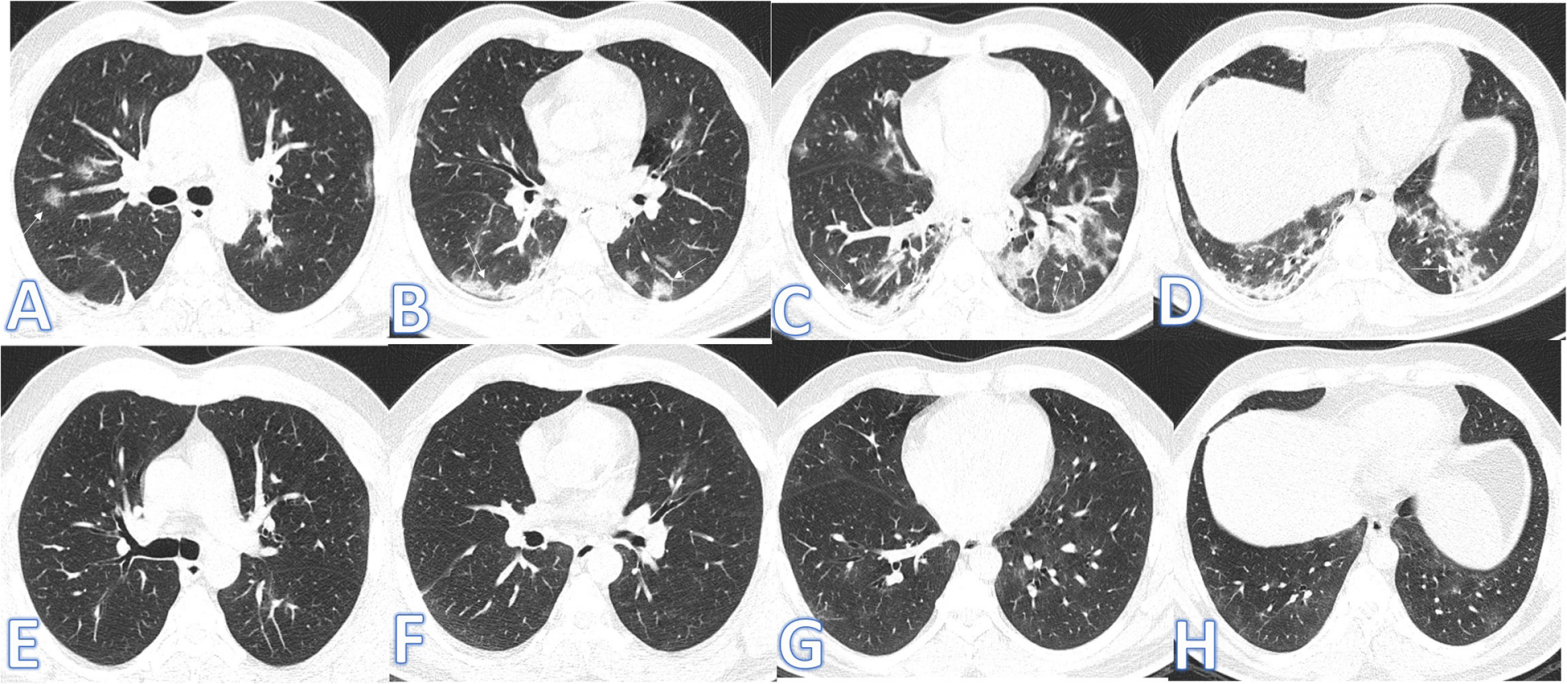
Thirty-eight-year-old male: he has no previous medical illness; CT severity score 7, consolidation/crazy-paving score 4. No ICU admission; laboratory results showed normal lymphocytes, serum ferritin, and d-dimer and high CRP and d-dimer. Steroid was not given; length of stay during hospitalization is 2days. Peak CT during admission (a–d) showed bilateral peripheral consolidation patches mainly in the lower lobes with rounded GGO (arrows). Follow-up CT 1month after discharge (e–h) showed total resolution of the previous lung changes
